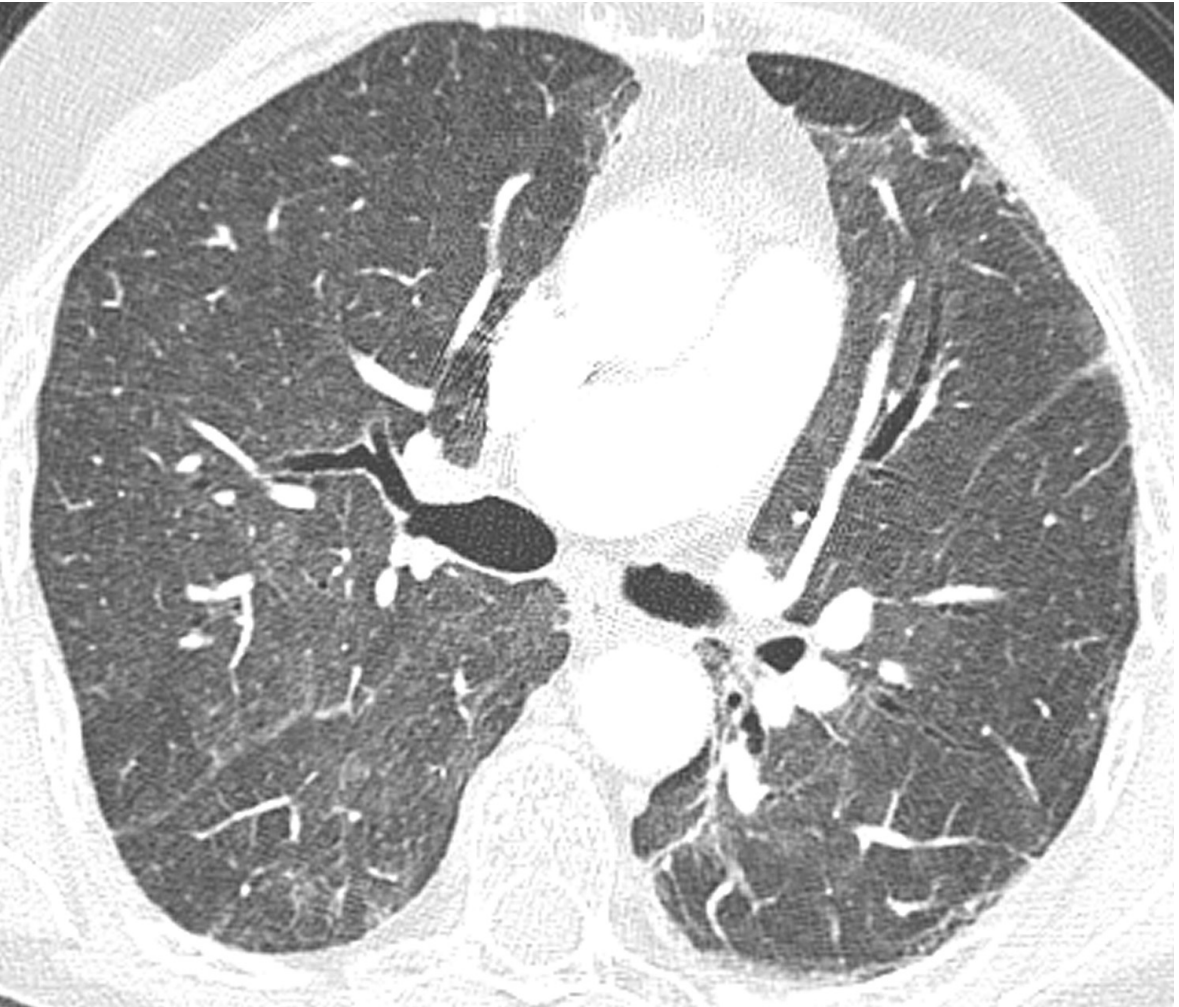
High-resolution transverse CT image shows extensive patchy ground-glass opacity throughout both lungs.
